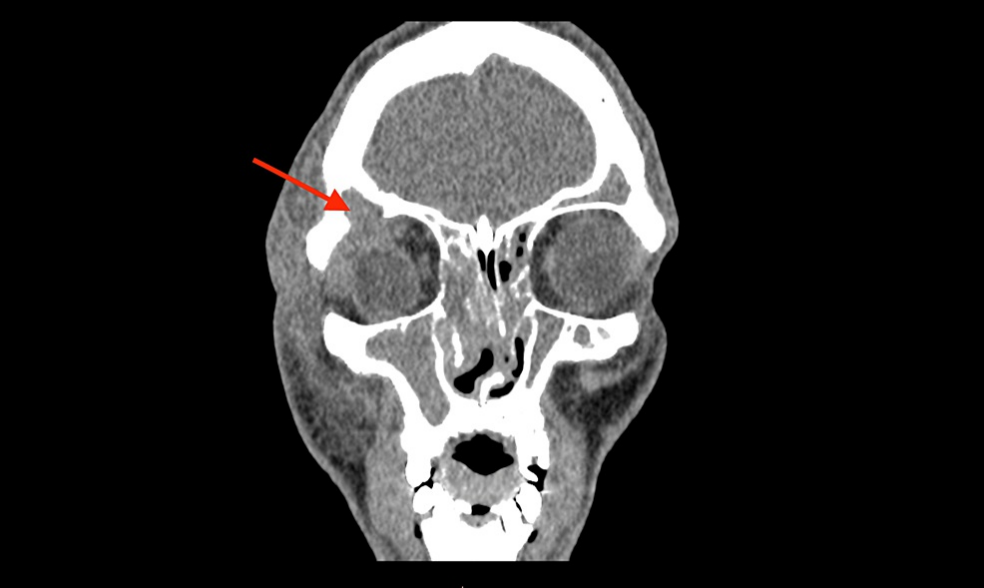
The same computed tomography (CT) slice with soft tissue windowing demonstrating soft tissue signal (the mucocoele) extending from the right frontal sinus to the superolateral aspect of the right orbit with consequent displacement of the orbit and associated more diffuse soft tissue swelling
